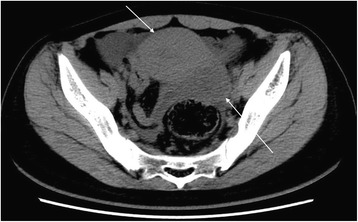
CT findings of ROCLC. The patient presented with persistent abdominal pain for a period of half a day with no clear cause; HCG test was negative. The longer arrow indicates the lack of any obvious cystic shadow in the left ruptured ovarian luteal cyst; the shorter arrow indicates the location of the uterus
